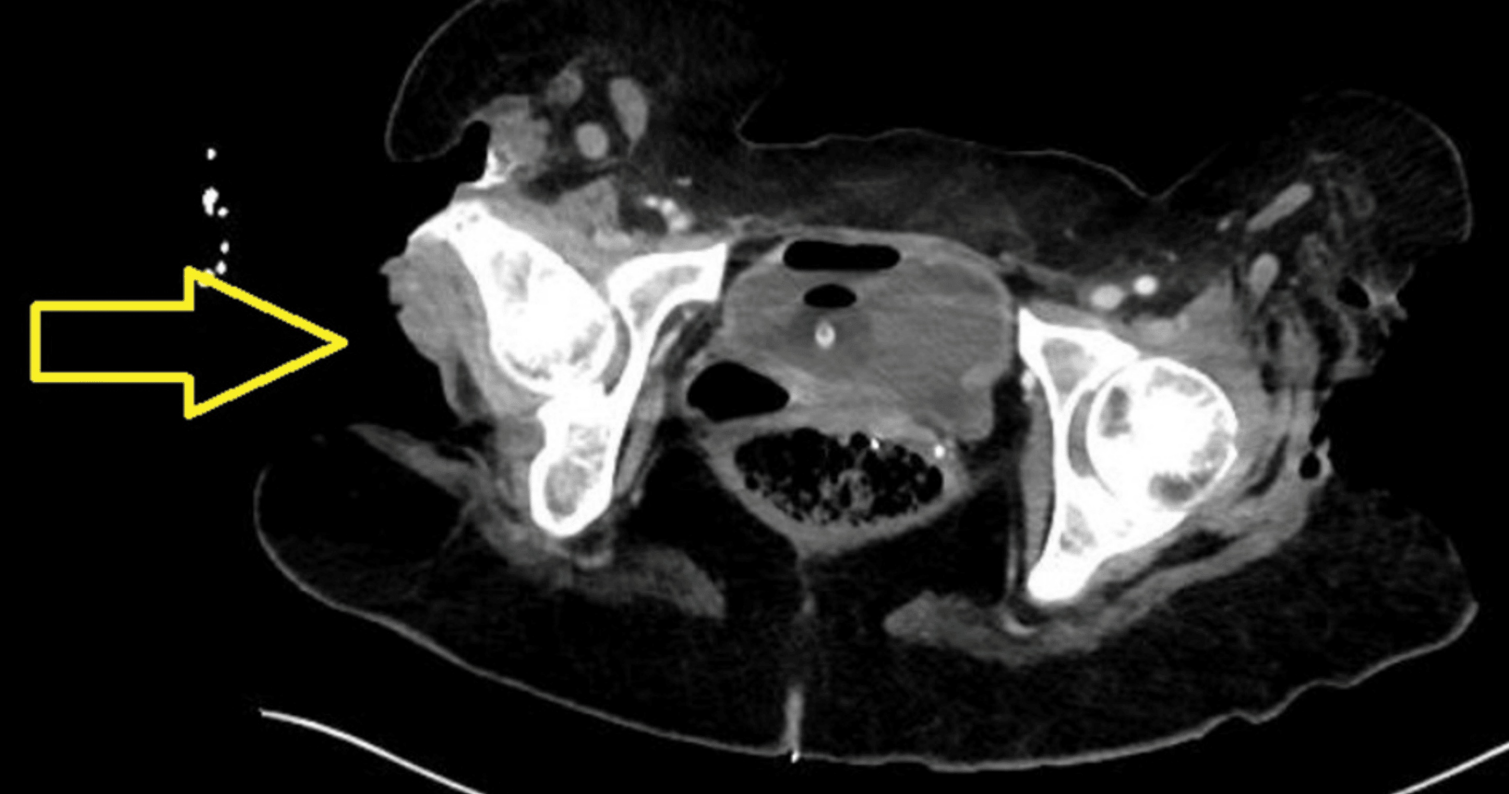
CT bilateral lower extremities with IV contrast revealing a large right wound of the right thigh extending from the iliac bone inferiorly to the right upper thigh approximately 21 cm in length (yellow arrow). There is air tracking superiorly along the gluteus musculature. There is exposed bone of the femur, with thinning and heterogeneity of the cortex of the exposed bone particularly at the greater trochanter. Minimal surrounding stranding of the soft tissues.
